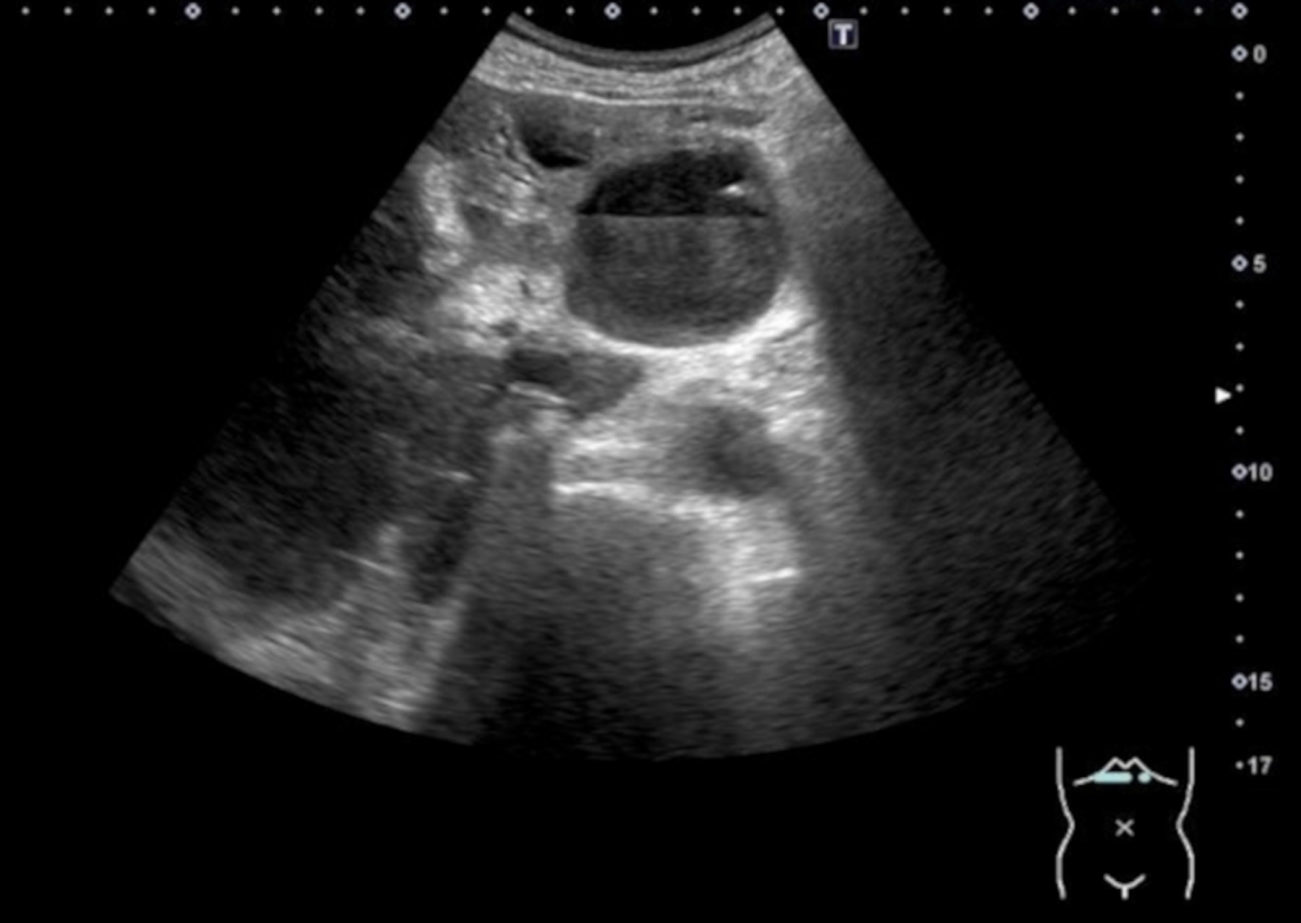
Abdominal ultrasonography revealed a hepatic cyst with infected fluid
